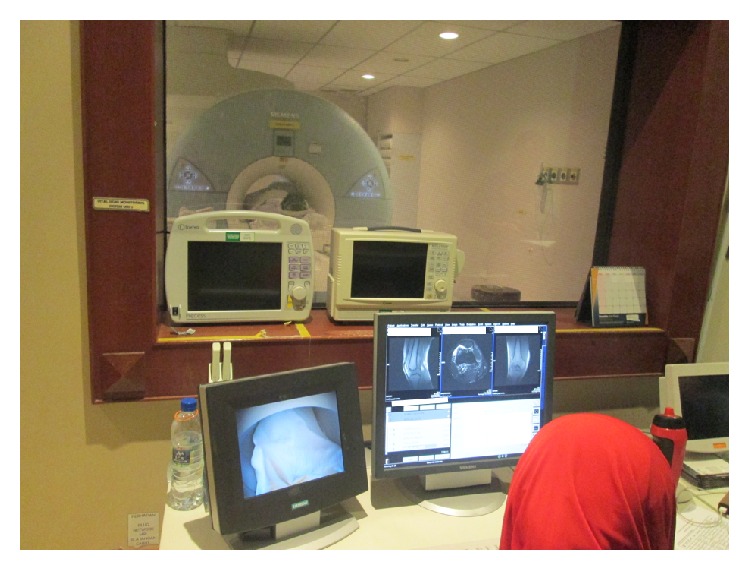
(a) MRI scanner at UKM Medical Center, Malaysia.
